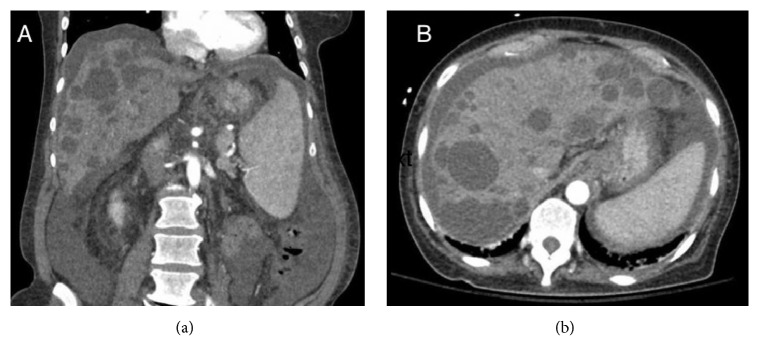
Computed tomography of abdomen and pelvis (coronal and axial scans) showing polycystic liver disease along with evidence of portal hypertension in form of splenomegaly and ascites.
